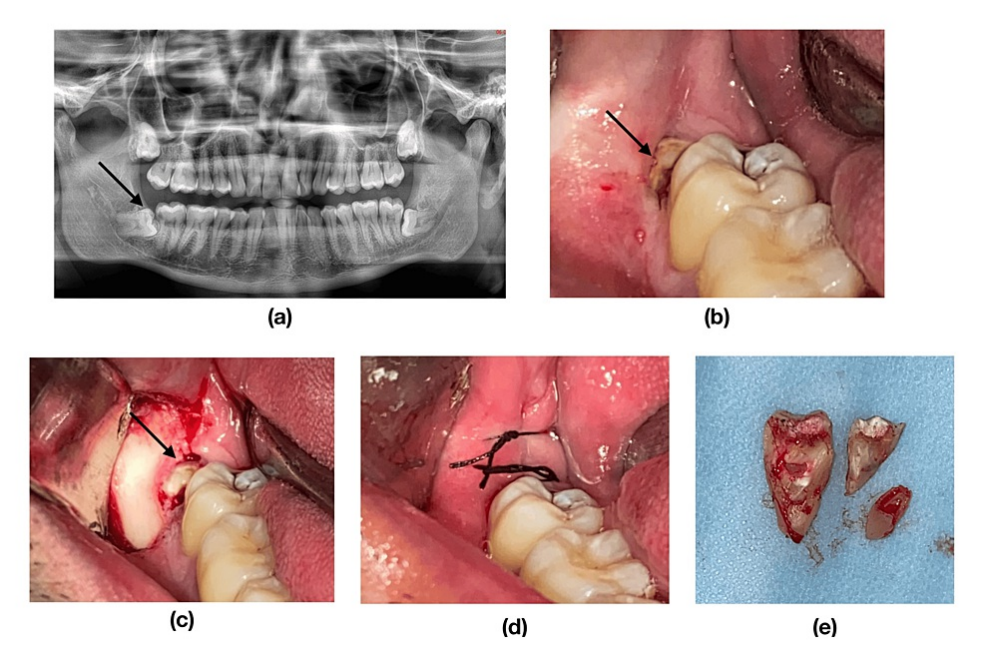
Surgical removal of impacted lower right mandibular molar tooth (a) Pre-operative orthopantomograph (black arrow indicates the impacted tooth) (b) Pre-operative intraoral photograph (c) Flap elevation (d) Closure (e) Extracted teeth
